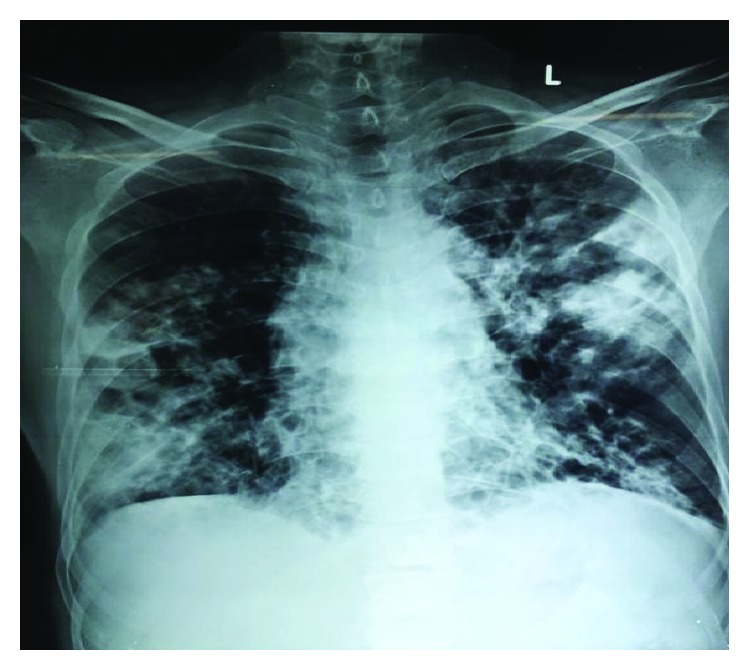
Chest x-ray showing bilateral midzone and lower zone consolidation.
